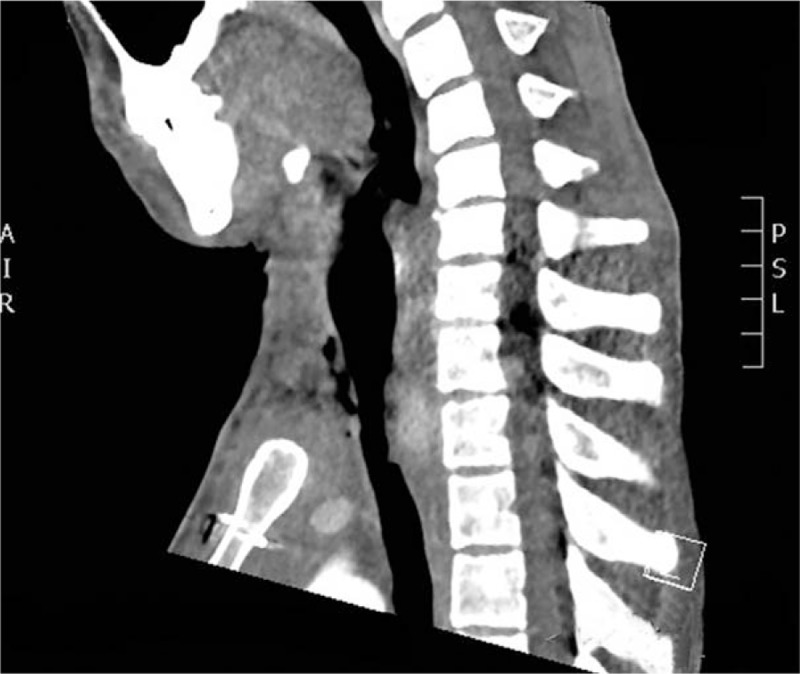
CT scan 8 days after operation.
